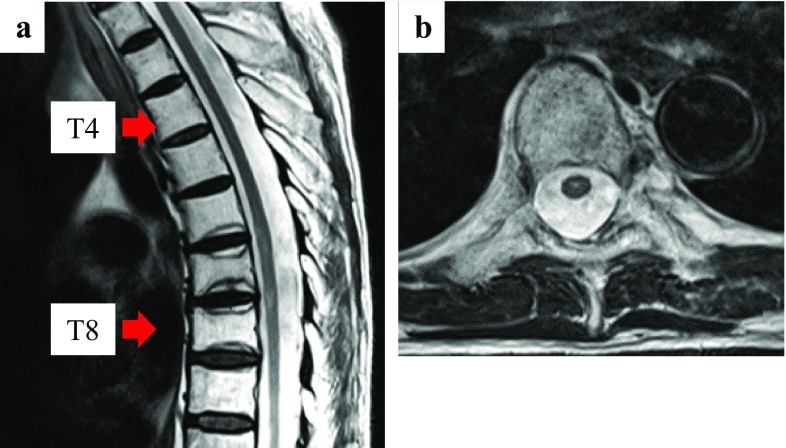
Magnetic resonance imaging 1 week after the starting date of steroid treatment. a Sagittal: T2 signal hyperintensity lesion between T5 and T8 spinal levels was unclear. b Axial: T2 signal hyperintensity lesion in the center of the spinal cord at T7 spinal level shrunk
